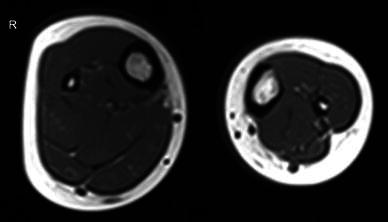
Axial T1-weighted spin-echo magnetic resonance image (TE-11 TR-656) sequences through the proximal aspect of both lower limbs. This demonstrates a normal superficial posterior compartment on the right. There is absence of most of the superficial compartment on the left, apart from atrophied lateral gastrocnemius and compensatory hypertrophy of the left deep posterior compartment and peroneal compartment
